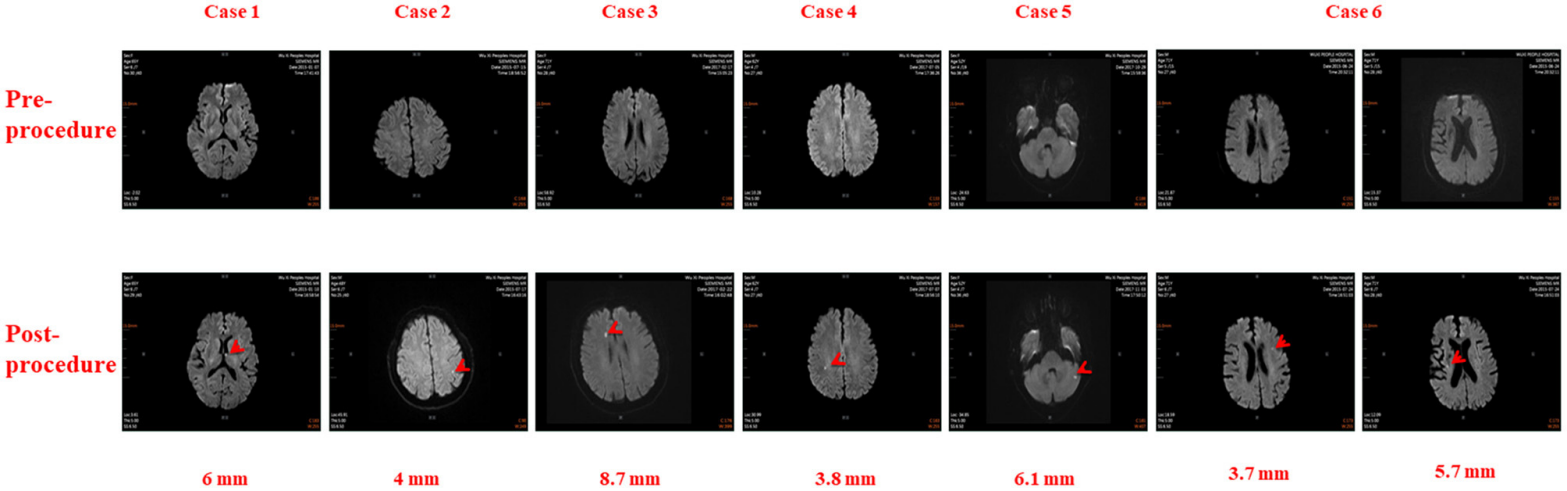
Cerebral Magnetic Resonance Images in six patients before and after Atrial Fibrillation Ablation using Magnetic Navigation System: Case 1: Left thalamus (6 mm), Case 2: Left parietal lobe (4 mm), Case 3: Right frontal lobe (8.7 mm), Case 4: Right parietal lobe (3.8 mm), Case 5: Left cerebellum (6.1 mm), Case 6: Left frontal lobe (3.7 mm) and Right corona radiata (5.7 mm).
